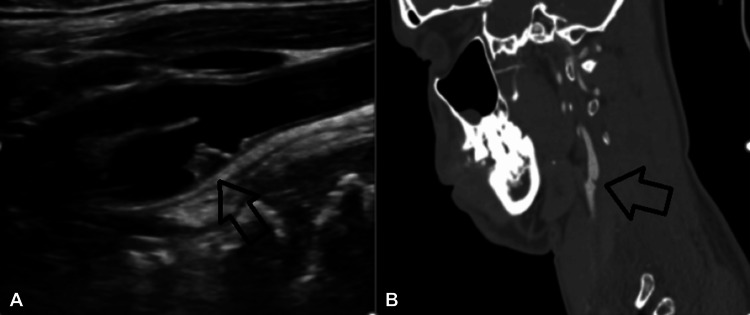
(A) Carotid DUS with an arrow pointing at a carotid web on the proximal ICA. (B) CTA showing healthy, non-calcified arteries, with an arrow pointing at focal narrowing of the proximal ICA DUS: duplex ultrasound; ICA: internal carotid artery; CTA: computed tomography angiography
